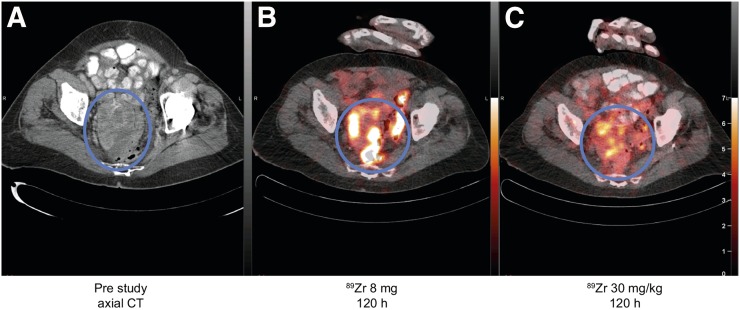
Images of patient 4: prestudy axial CT scan (A), ^89^Zr-GSK2849330 PET/CT after 8-mg total mAb dose at 120 h after injection (B), ^89^Zr-GSK2849330 PET/CT after 30 mg/kg total mAb dose at 120 h after injection (C). Circles highlight large metastasis in pelvic region. Both ^89^Zr-GSK2849330 PET images are normalized to same intensity.
